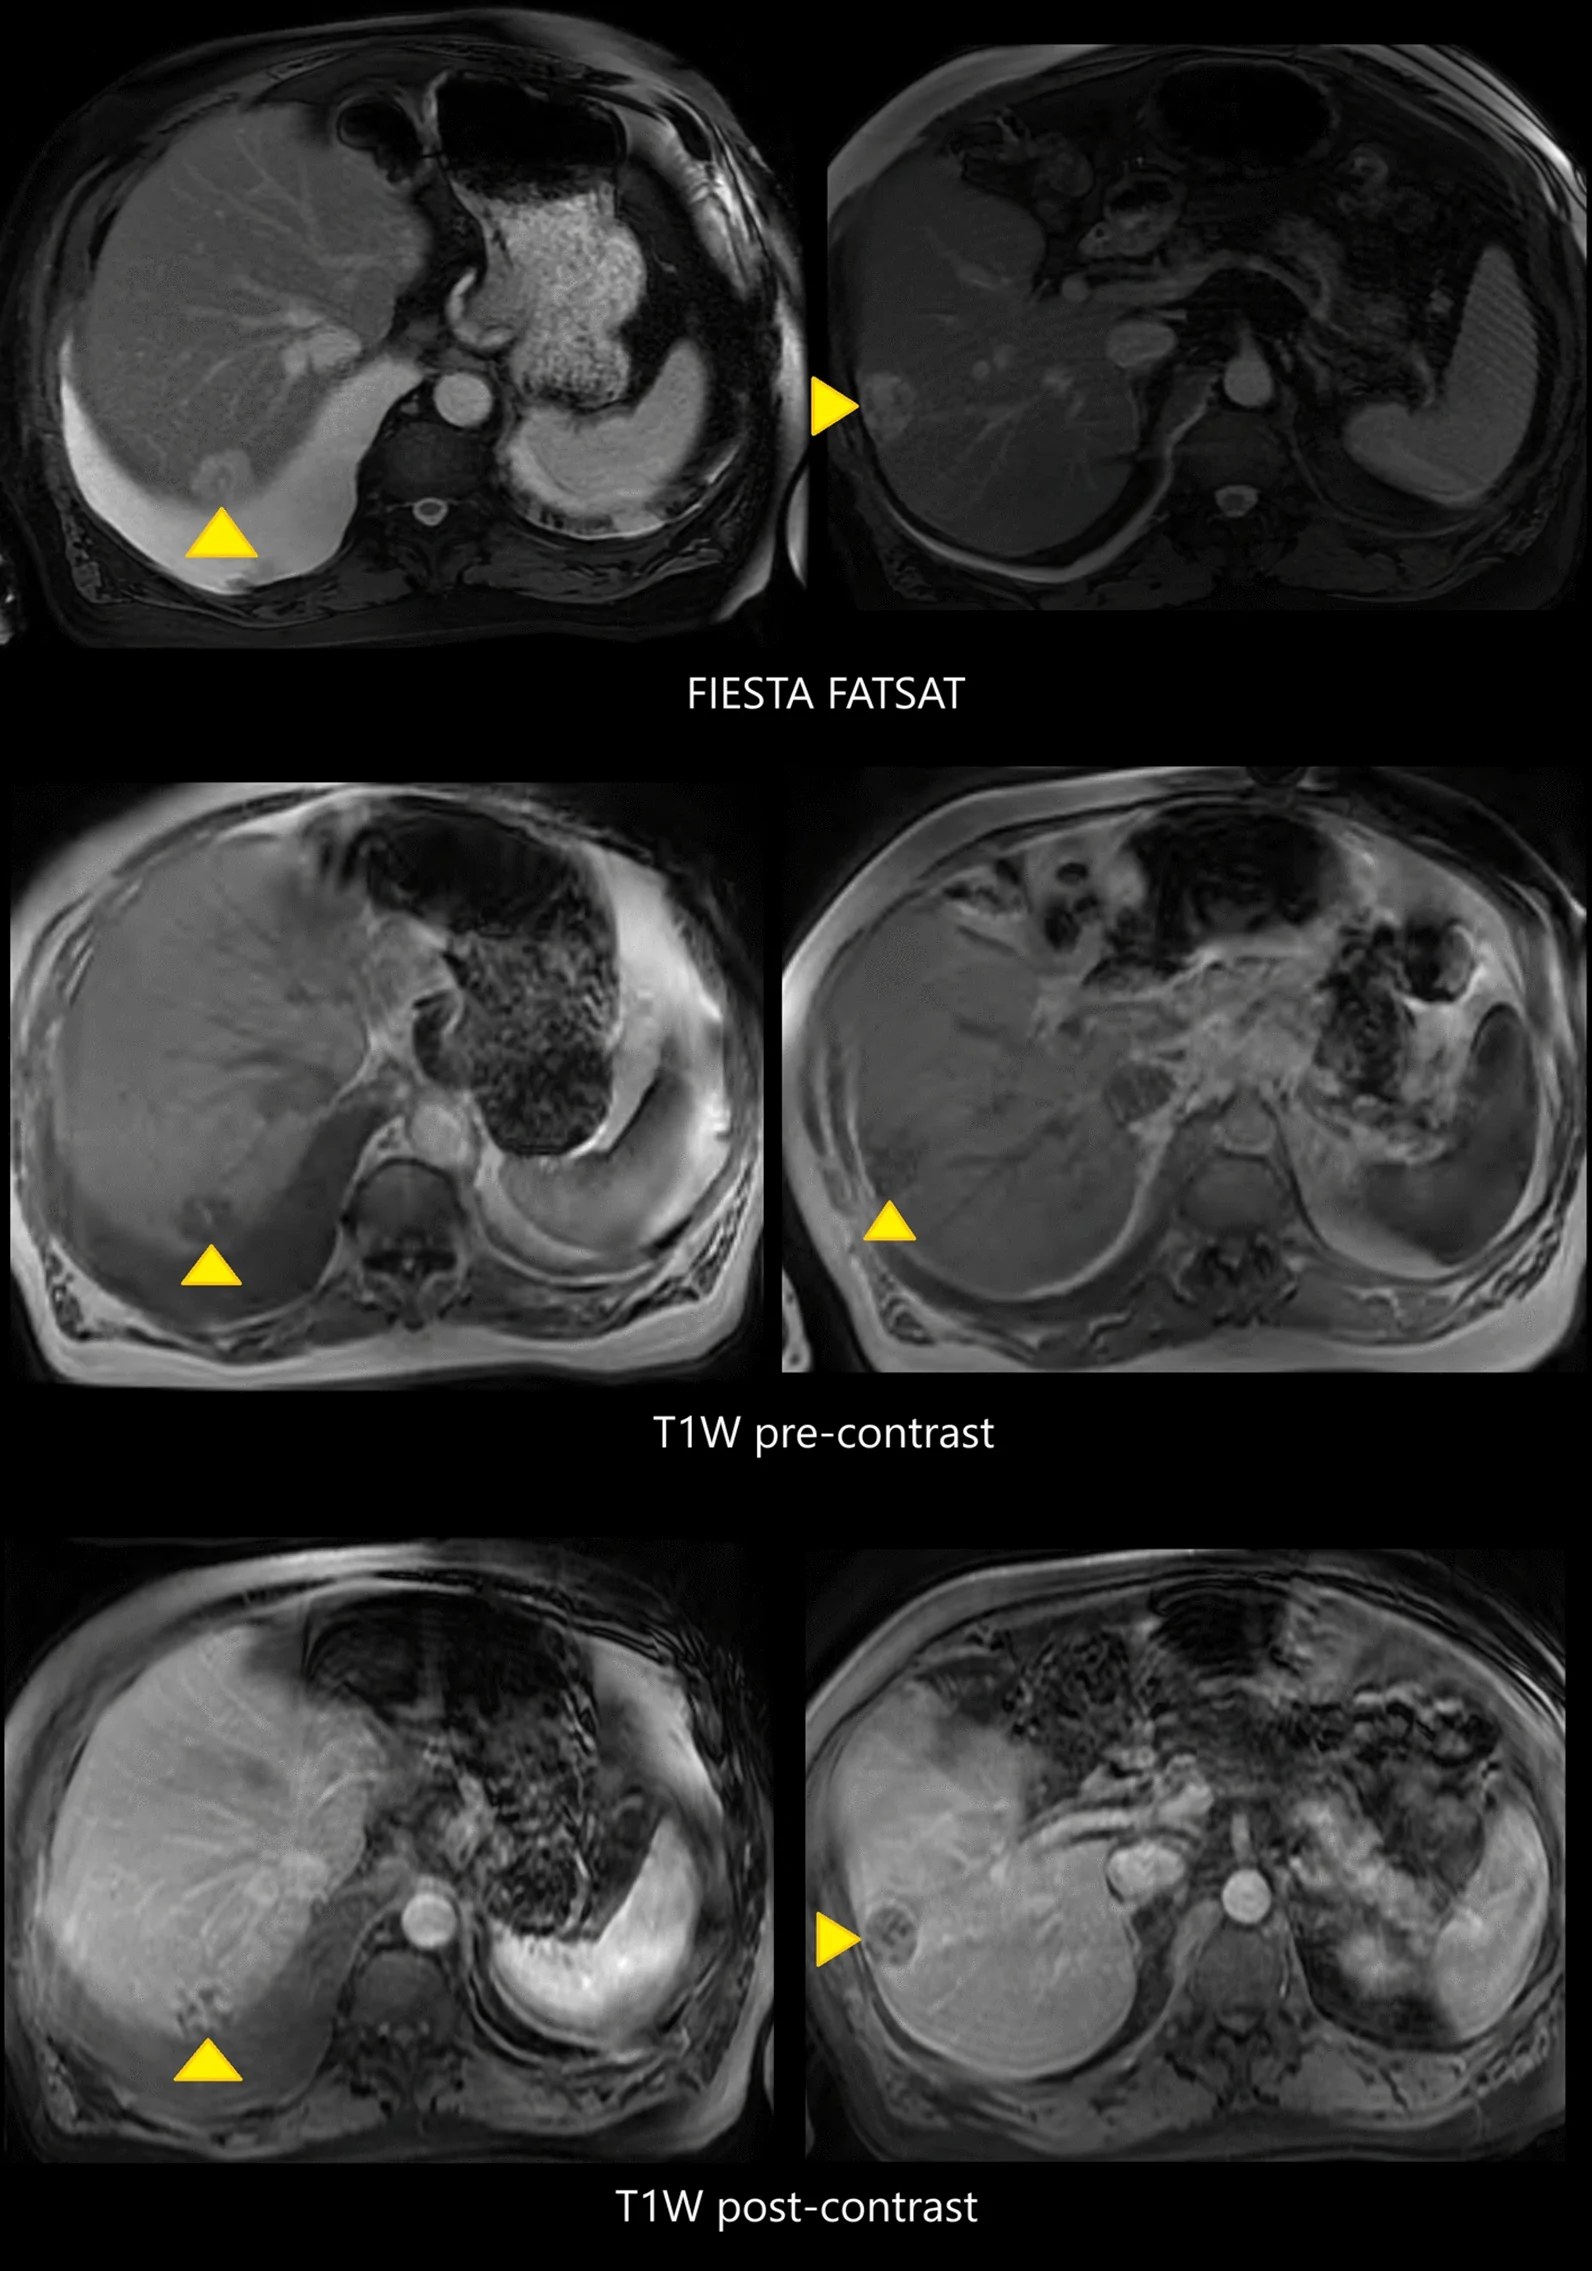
Magnetic resonance imaging of the liver demonstrating liver masses (arrowheads). Axial slices of magnetic resonance imaging showing two liver masses (arrowheads), which are hypointense on T1-weighted imaging and hyperintense on Fat saturation sequences. *FATSAT* = Fat saturation; *FIESTA* = fast imaging employing steady-state acquisition; *T1W* = T1-weighted imaging
